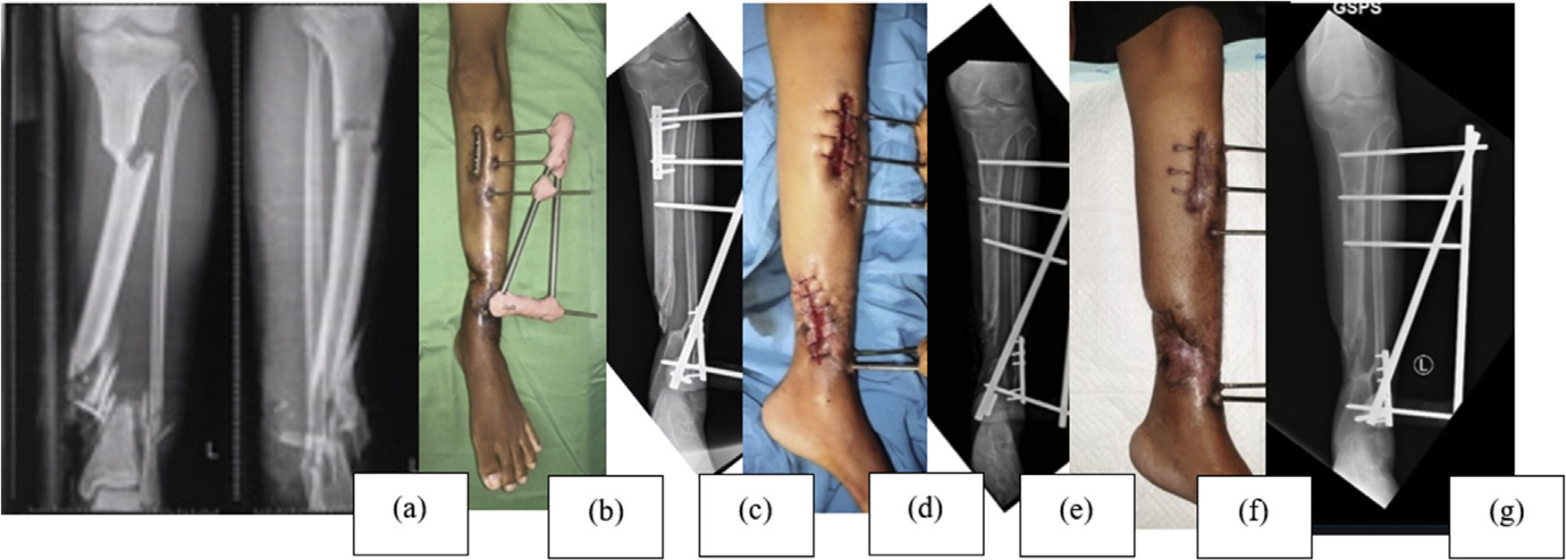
Case 1; a 23-year-old male with infected non-union of left distal tibial shaft. (a) Initial anteroposterior and lateral X-ray of the tibia. (b) Anterior preoperative view of the left leg – exposed sequester. (c) Preoperative anteroposterior X-ray of the left leg. (d) Anterior postoperative view of the leg. (e) Postoperative anteroposterior X-ray of the left leg. (f) Anterior view of the left leg, six months postoperatively – wound was healed. (g) Anteroposterior X-ray of the left leg, six months postoperatively – bridging bone formation.
